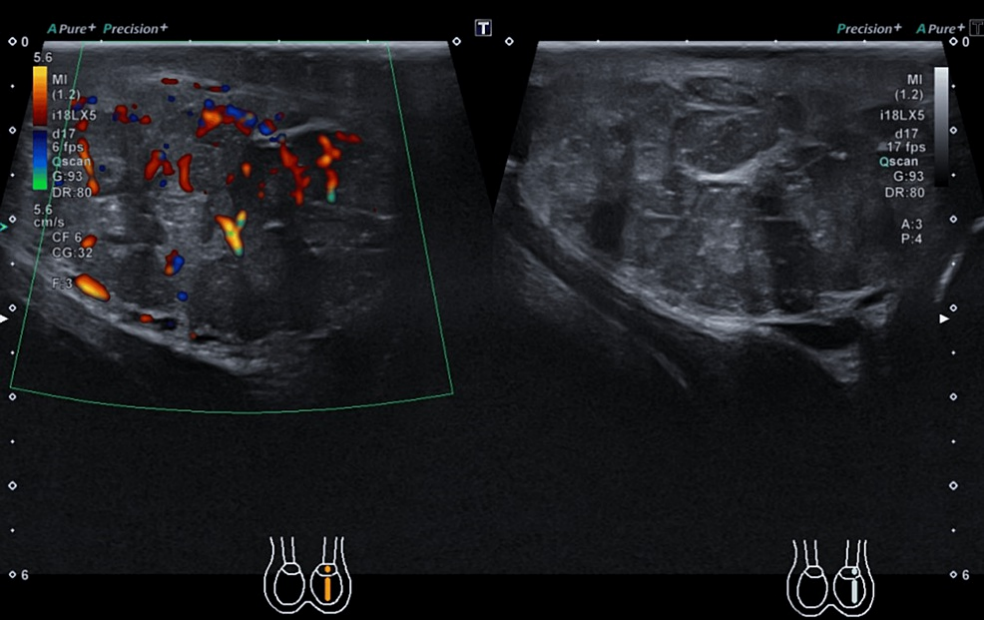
Ultrasound of the scrotum.
